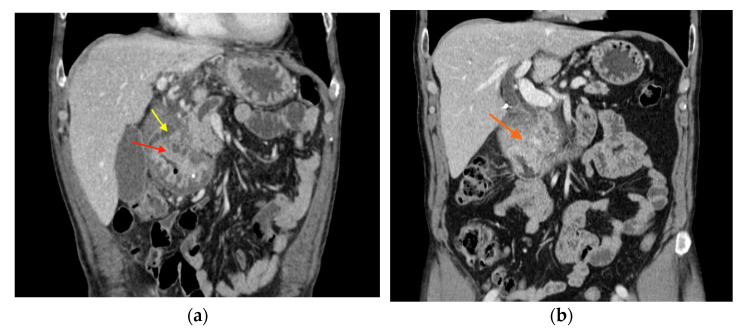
(a) CT image in a patient with non-tumor-like GP (yellow arrow: intramural cysts; red arrow: duodenal wall thickening). (b) CT image in a patient with tumor-like GP (orange arrow: tumor-like aspect of the head of the pancreas).
